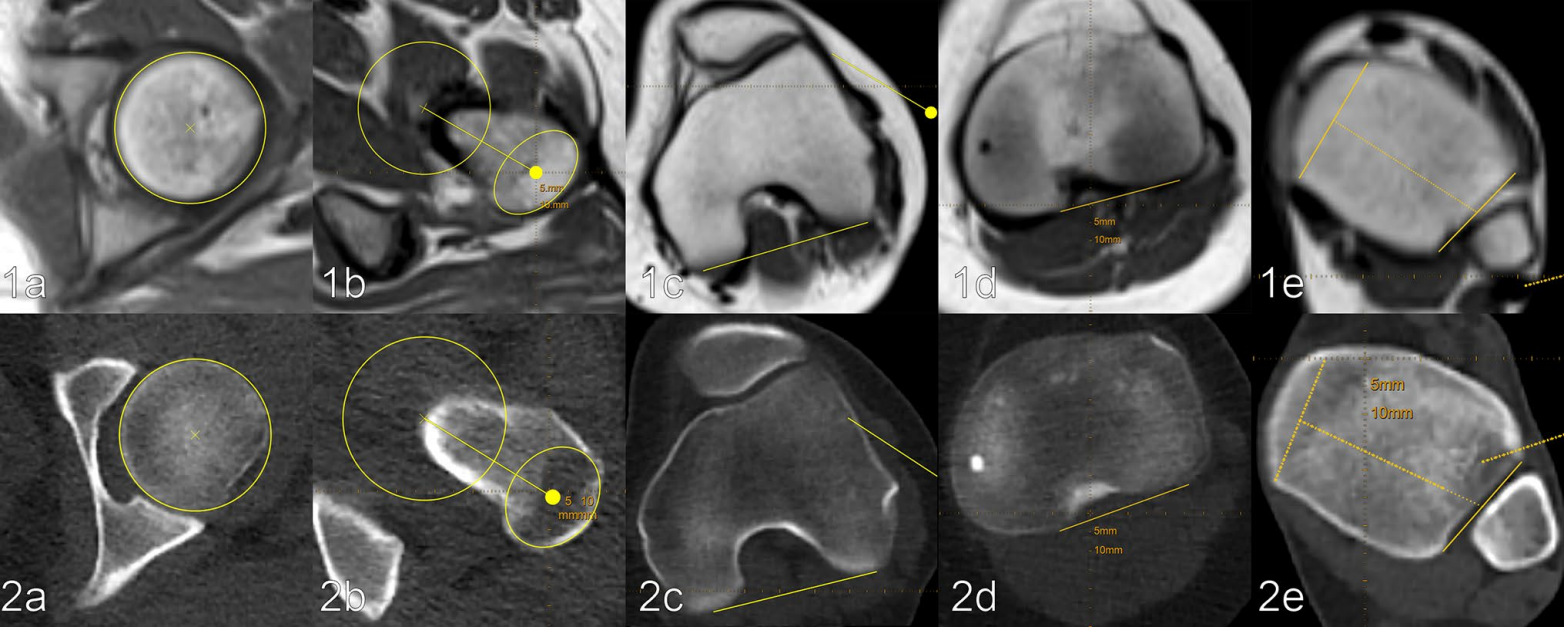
Comparison of CT and MRI for torsion measurement of the lower limb in an exemplary patient (female, 18 years, BMI = 24 kg/m^2^), scanned in the same position. The figure depicts exemplarily the captured images as well as the drawn reference lines. Number (1) depicts MRI images and number (2) CT images, respectively, for: a hip centre, b centre of the greater trochanter, c posterior margin of the femoral condyles, d posterior margin of the tibial plateau, e line through the centre of the medial malleolus and centre of the fibular incisura of the tibia
